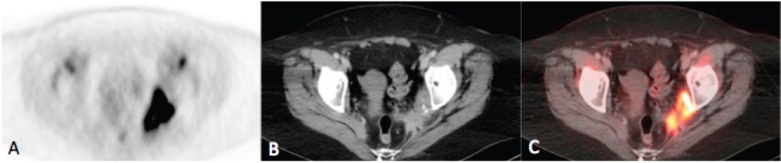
Axial PET(A), CT (B) and fusion (C) images of the pelvis demonstrating intense uptake (SUVmax = 21.9) within an irregularly marginated soft tissue mass at the pelvic sidewall containing punctate calcifications, consistent with the biopsy-proven metastatic squamous cell carcinoma.
